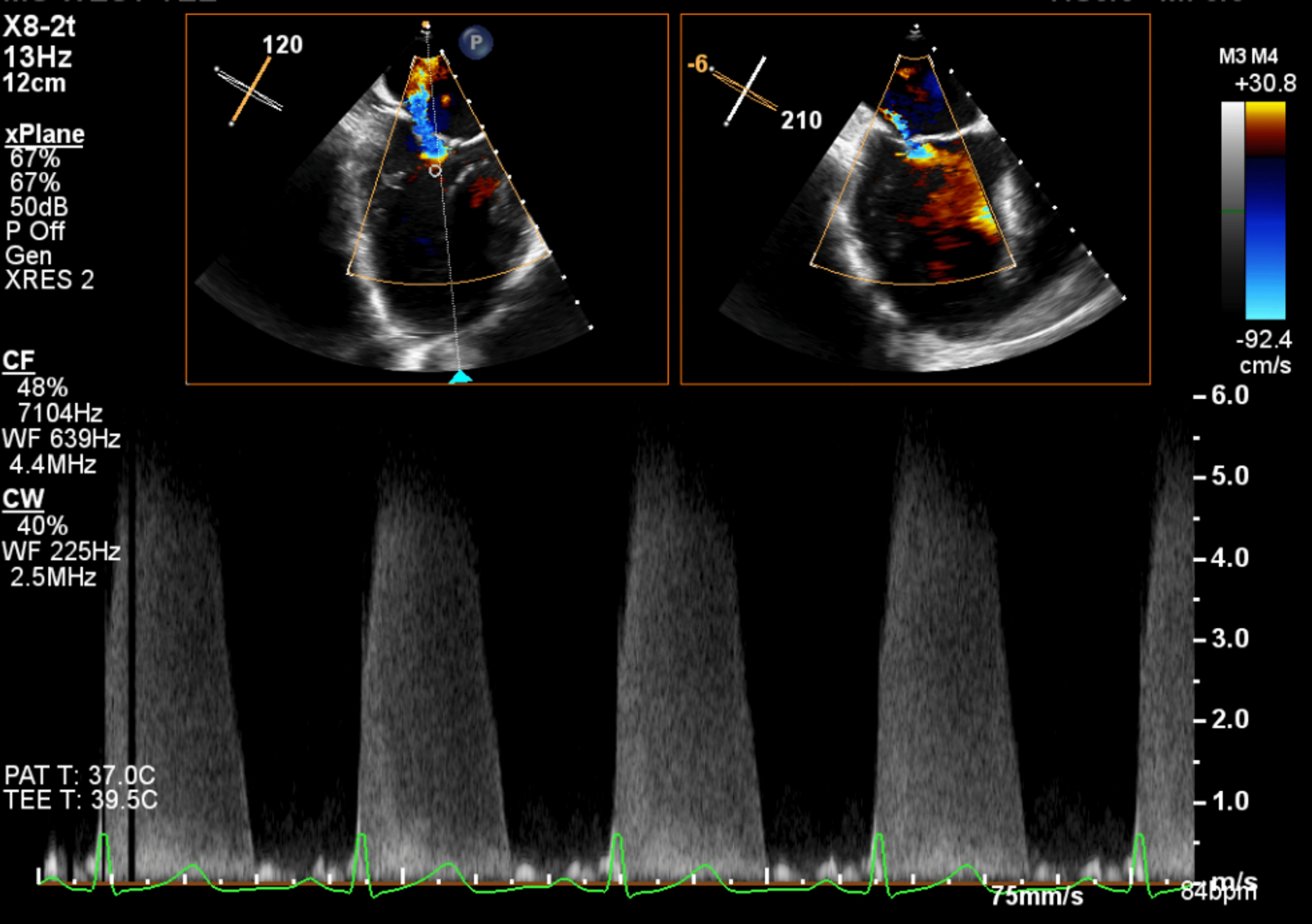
Continuous-wave Doppler evaluation of mitral regurgitation.
